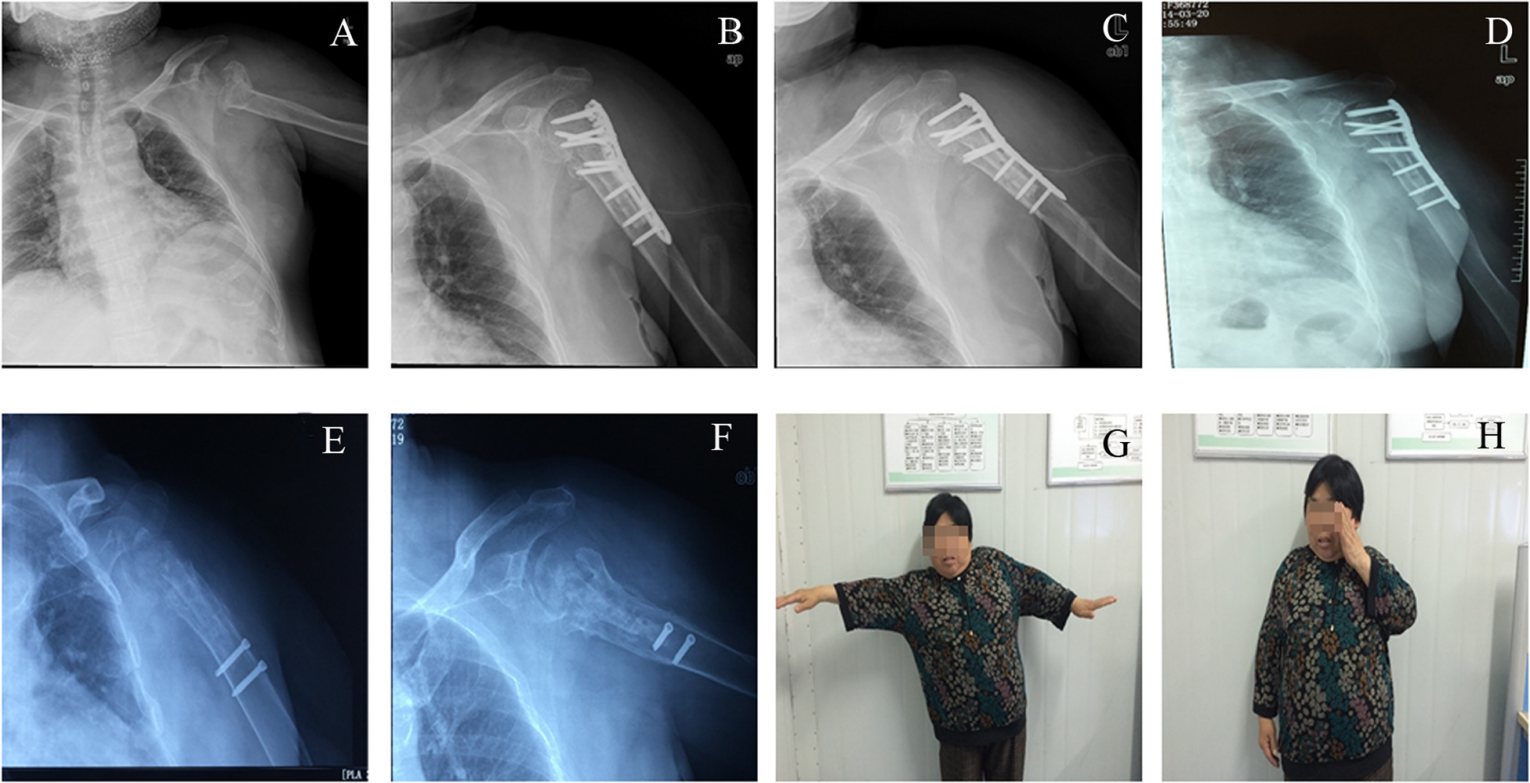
One case develops AVN and screw cutout but gets satisfactory outcomes after the implant is being removed. a X-ray film before surgery. b X-ray film after surgery. c X-ray film 3 months after surgery. d X-ray film 12 months after surgery. e, f X-ray film 30 months after surgery. g, h Function of patient’s upper arm
